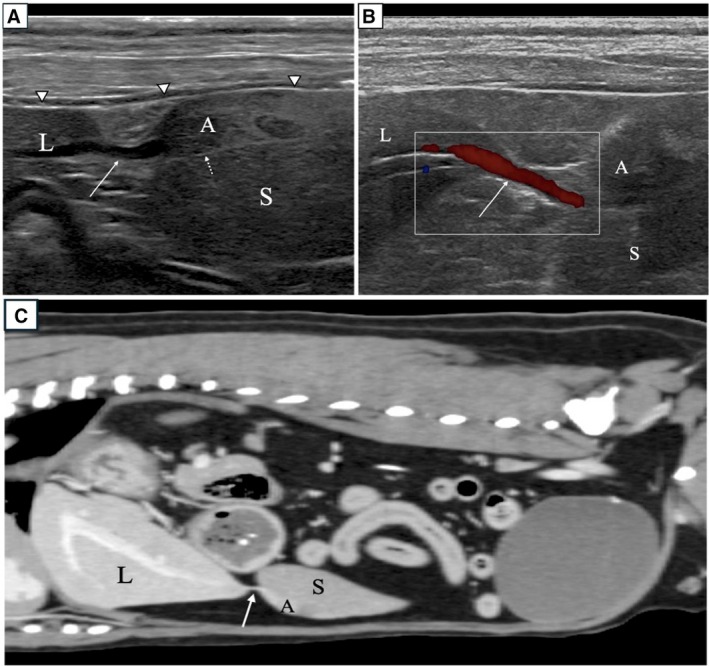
In all images, accessory liver lobe is labelled as “A”, the spleen as “S”, the liver as “L” and the pedicle as an “arrow”. (A) Dorsal ultrasonographic view of the accessory liver lobe appearing connected to the liver by a pedicle; the structure is located between the left abdominal wall (arrowheads) and the spleen, clearly separable from the splenic tail (dashed arrow). (B) Colour Doppler ultrasound image depicting the vessel within the pedicle. (C) Sagittal post‐contrast CT image showing the vascular pedicle between the liver and the accessory liver lobe.
